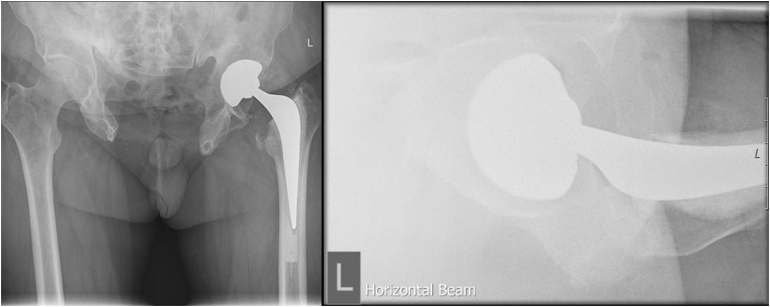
Postoperative anteroposterior and lateral x-rays of left hip total replacement
